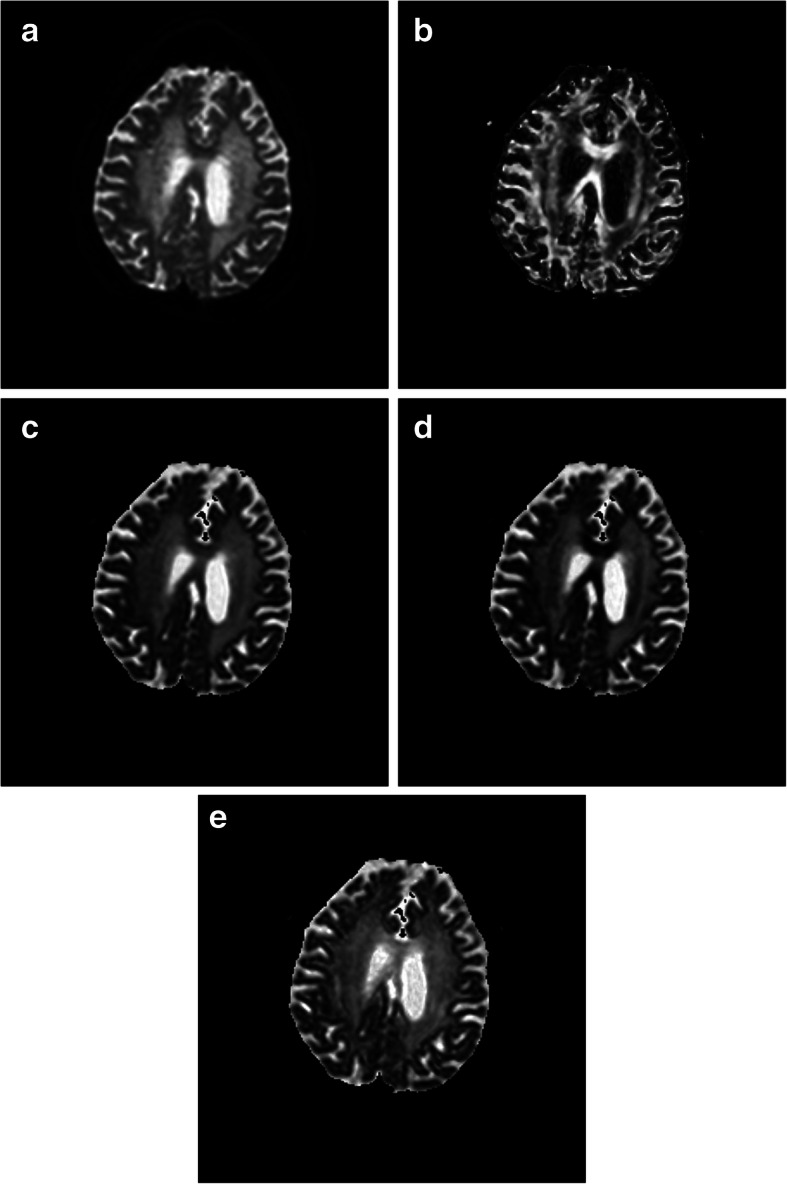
The b0 of the DWI (a) of a 83 years old female showed WMH (Fazekas scale 3) in the pFL, pOL and dCO. FA (b) of WMH in the pFL are lower than that of pPL and dCO. MD (c) and RD (d) of WMH in the pFL are higher than that of pPL and dCO. AD (e) of WMH in the pFL are close to that of pPL and dCO
